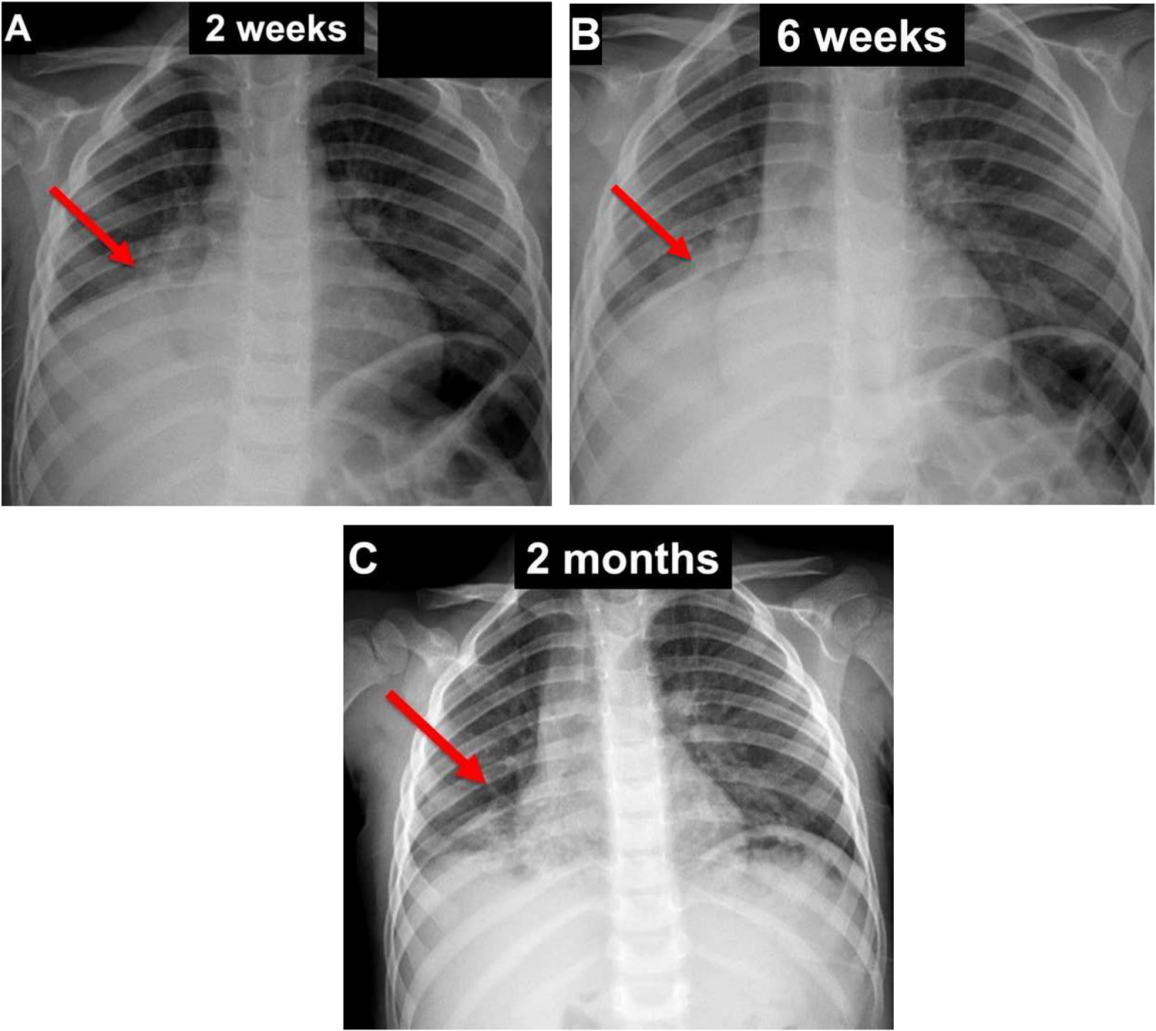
Sequential frontal chest radiographs approximately (A) 2 weeks, (B) 6 weeks, and (C) 2 months from initial symptoms show a relatively unchanged right middle and lower lobe consolidation over the course of 2 months.
